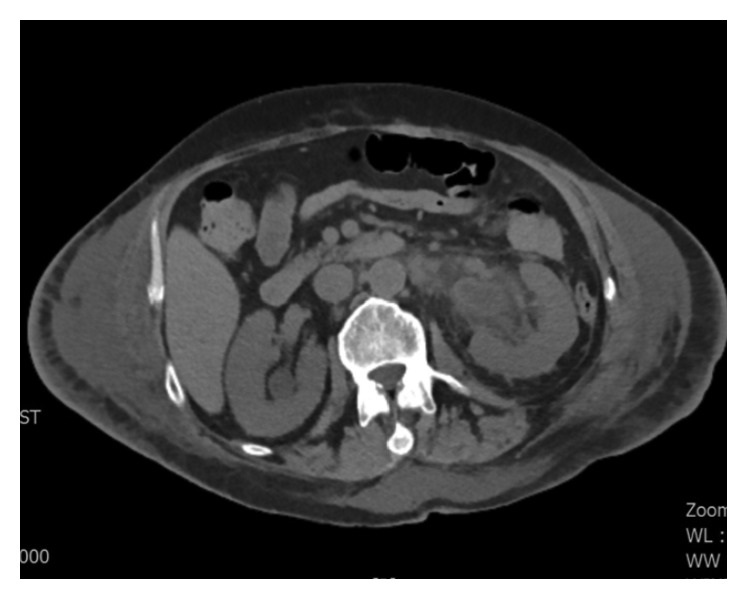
CT abdomen (transverse view) shows bilateral hydronephrosis.
